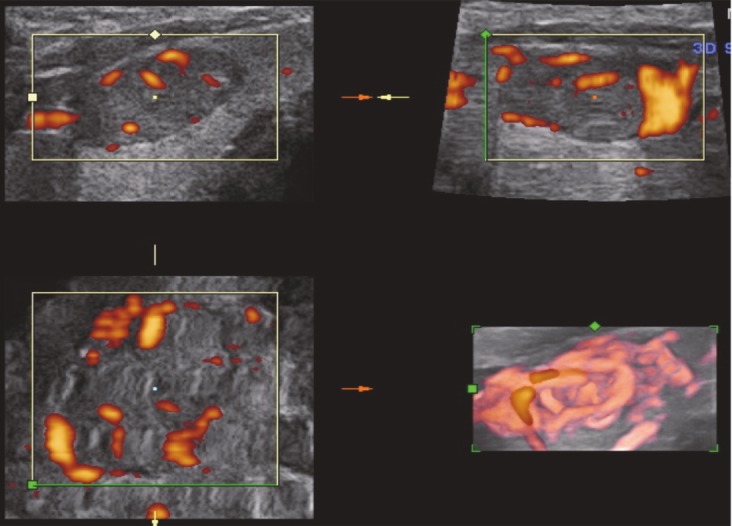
Sonogram showing 3D power Doppler acquisition in the axial plane using 3D power Doppler ultrasound. The power Doppler box was positioned so as to cover the entire thyroid nodule. The widest scanning angle (29°) including the whole thyroid nodule was used.
